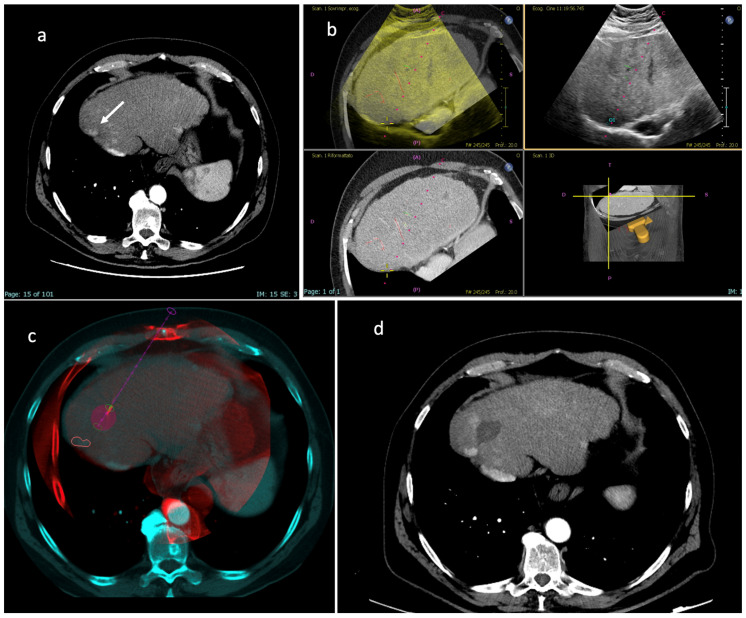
Missed target; (a) Axial CT image in the arterial phase showing a hypervascular nodule in S8, postero-lateral to an ablation volume from previous treatment, consistent with recurrent disease (white arrow). (b) US fusion imaging is performed by registrating pre-procedural CT images to real-time US images, and the antenna is placed within the target tumor. (c) CBCT fusion imaging indicates that the antenna and the predicted ablation volume (purple) are distant from the tumor (red contour), differently from what is suggested by US fusion imaging. Since the registration quality between US real-time images and pre-procedural CT images appeared good, the operator decided to ablate without repositioning the antenna. (d) CT axial image in the arterial phase at 1-month follow-up resulted comparable to intraprocedural CBCT fusion imaging, demonstrating that the nodule was missed.
